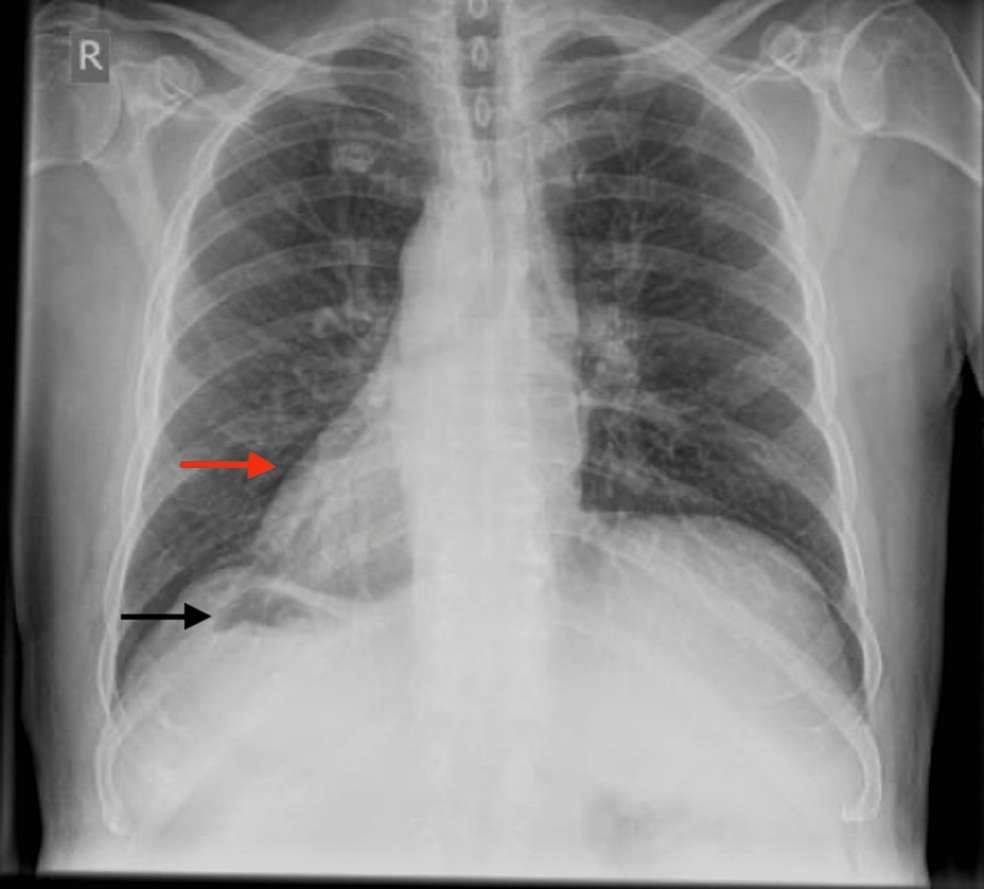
Chest X-ray shows dextrocardia (red arrow) and gastric bubble (black arrow) on the right side of the abdomen
